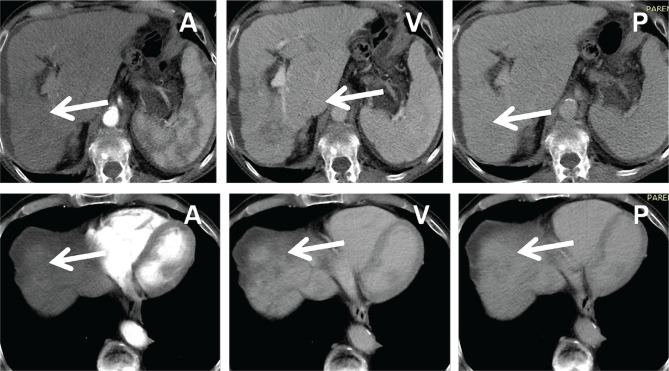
CT scan showing multiple heterogeneous small liver masses A – arterial phase; V – venous phase; P – Portal phase.
